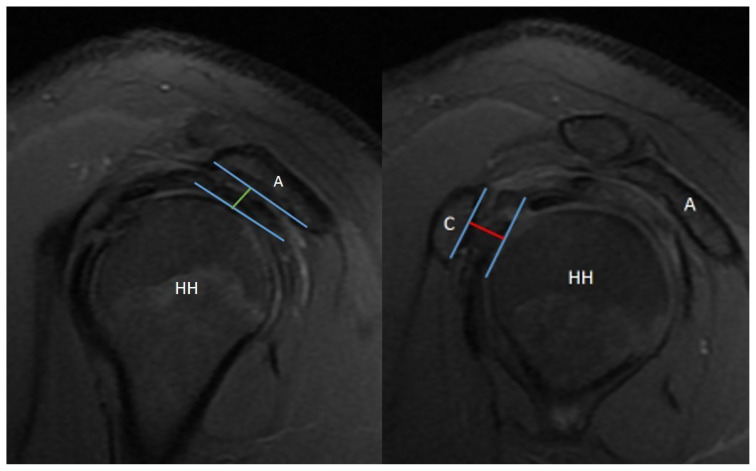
Acromiohumeral distance (AHDsag-green line) and coracohumeral distance (CHDsag-red line) on sagittal oblique sections of magnetic resonance imaging scans.
